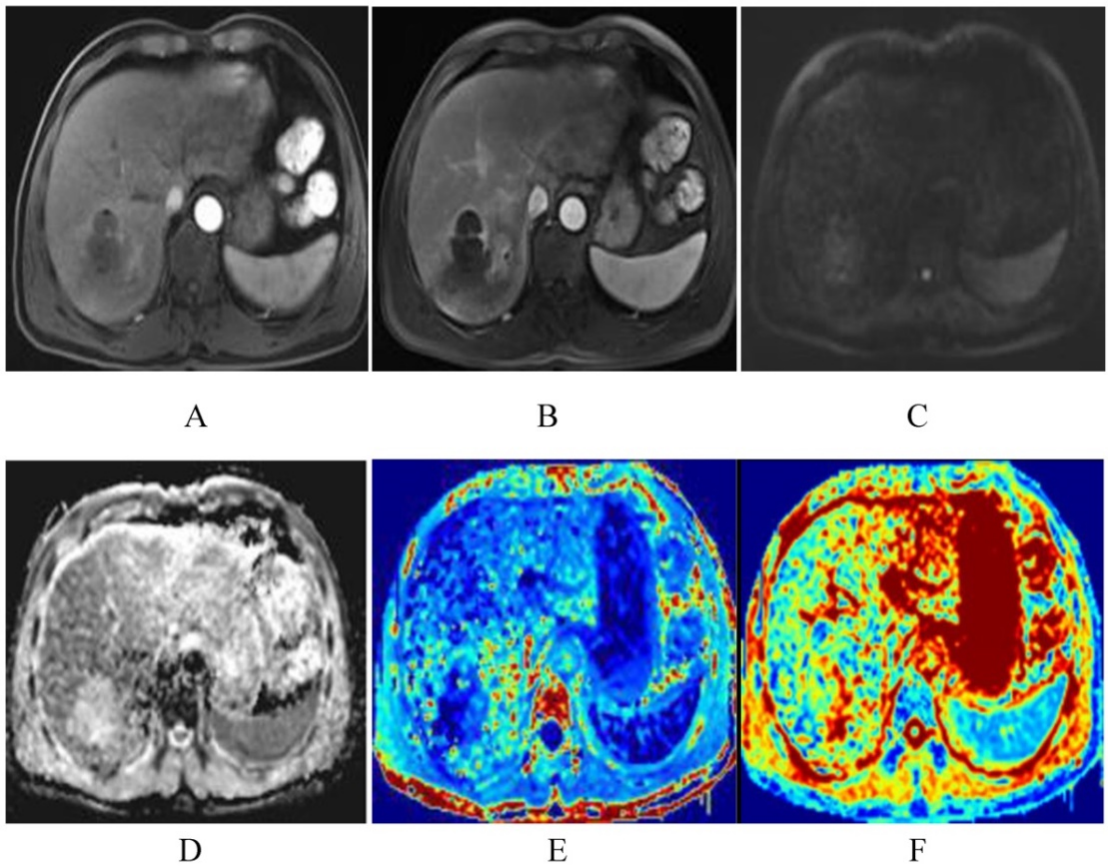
The same patient with Fig. 2 after TACE. The degree of lesion enhancement is significantly reduced and DWI signal is decreased (A-C). ADC and MD map after TACE show higher signal intensity than the residual tumor. The values for the lesion are 1.55×10^-3^mm^2^/s and 2.15×10^-3^mm^2^/s respectively (D-E). Fig. F is the kurtosis map, showing lower signal intensity than the residual tumor. The value for the lesion is 0.45.
